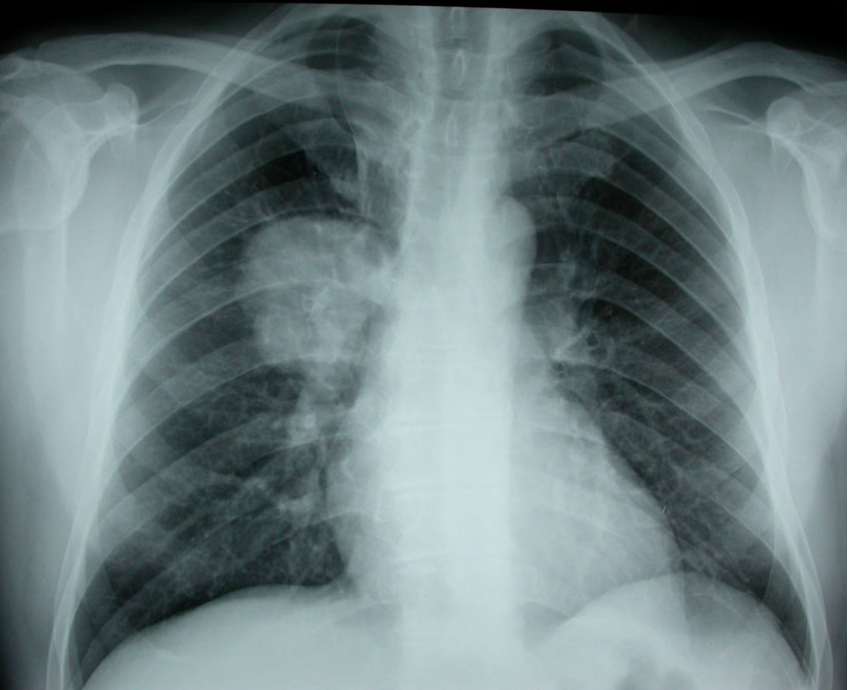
Plain chest radiograph showing a large homogenous tumour-like opacity in the upper lung zone near the right hilum.
